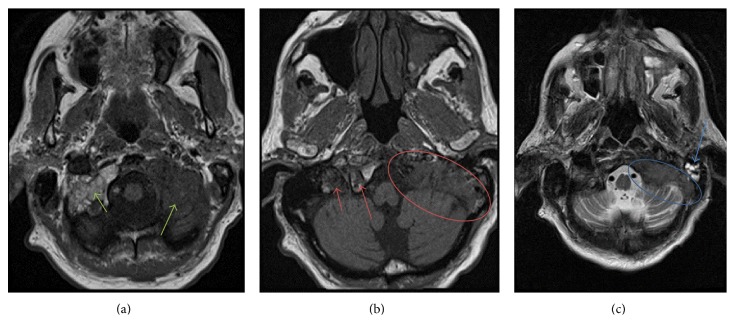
MRI scan of the head, 7/9/2014. (a) Axial T1 image shows a large posterior skull base mass. Note the normal bright fatty marrow (short green arrow) compared to darker signal from the mass (long green arrow). (b) Axial T1 image shows the mass (red oval) involving both the jugular foramen and the hypoglossal canal. Note the normal position of the contralateral jugular foramen (short red arrow) and the hypoglossal canal (long red arrow) for reference. (c) Axial T2 image shows involvement of the petrous temporal bone (blue oval) extending into the posterior inferior mastoid air cells, with bright reactive mastoid fluid (blue arrow).
